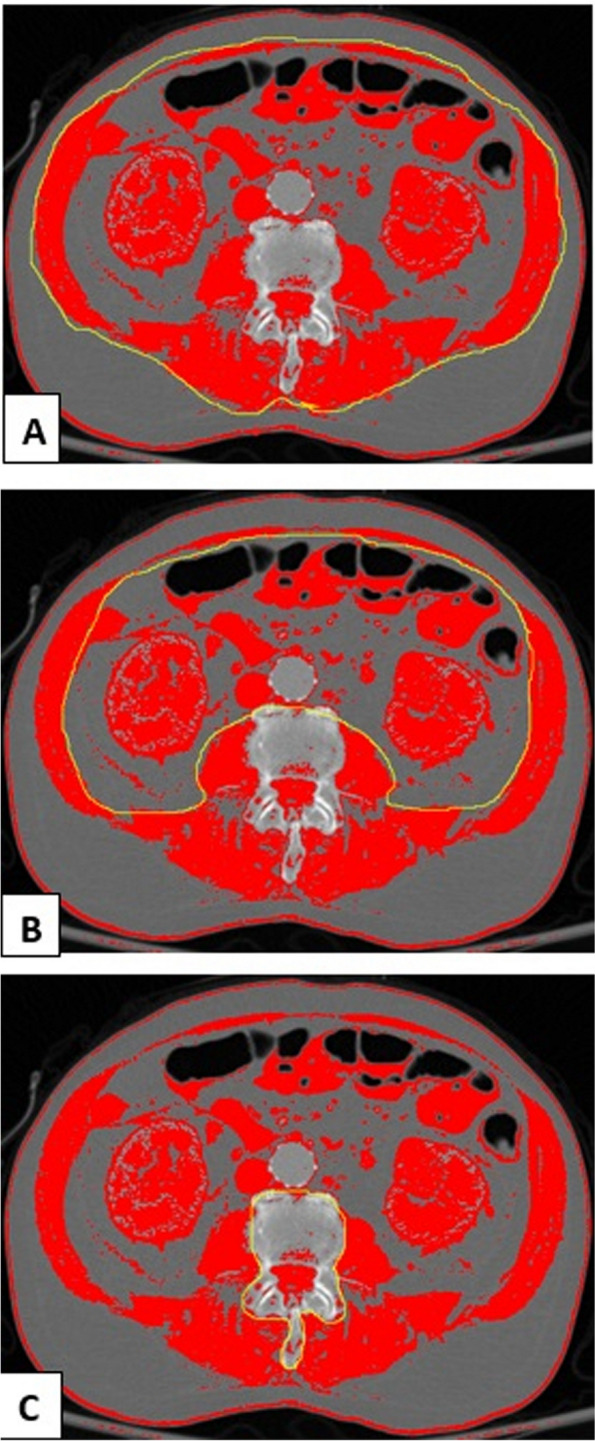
Measuring skeletal muscle area on L3 axial slice Computer-Tomography (CT) Image, Using the National Institutes of Health ImageJ. Radio density thresholds were selected to correlate with those of skeletal muscle, with lower and upper thresholds of − 29 and 150 Hounsfield units, respectively. A Outer perimeter of the abdominal muscles is first delineated (yellow tracing) and area calculated, B The process is repeated for the inner perimeter of abdominal muscles (yellow tracing), C Vertebral area is further excluded (yellow tracing). The difference between measures equates to the area of skeletal muscle at the L3 level
